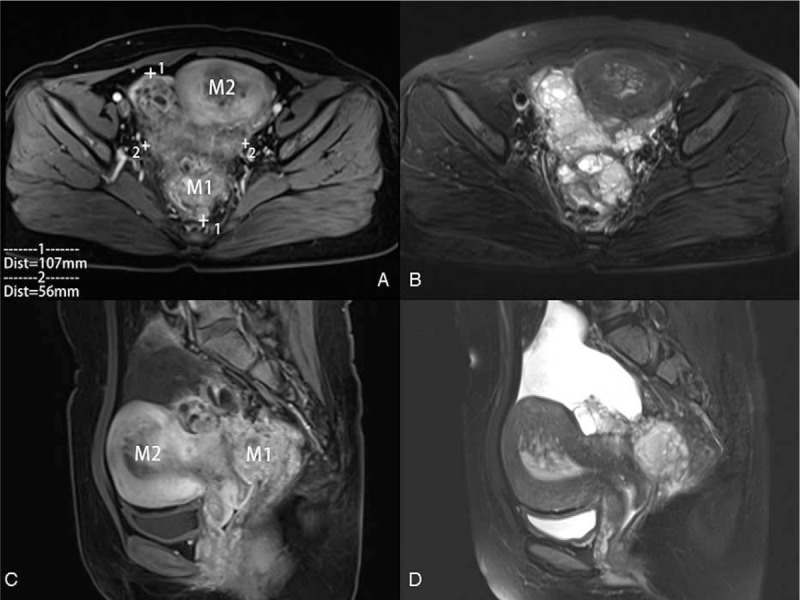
Gadolinium-enhanced T1-weighted images axial (A) and sagittal (C), T2-weighted image axial (B), and sagittal (D) of pelvic MRI showing an irregular mass of the right adnexal (M1) of about 56∗107 mm^2^ with low-signal and partial high-signal and without distinct border with the rectum; an uterine cavity's ill-defined mass with heterogeneous signal (M2), rooting in the myometrium of the posterior wall of the uterus, without distinct border with the uterine wall. MRI = magnetic resonance imaging.
